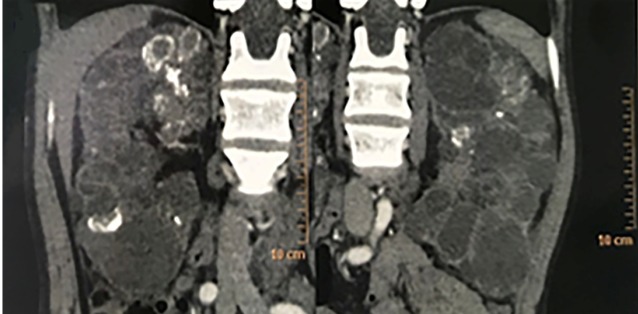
Coronal images of the patient’s abdominal CT scan showing enlarged
